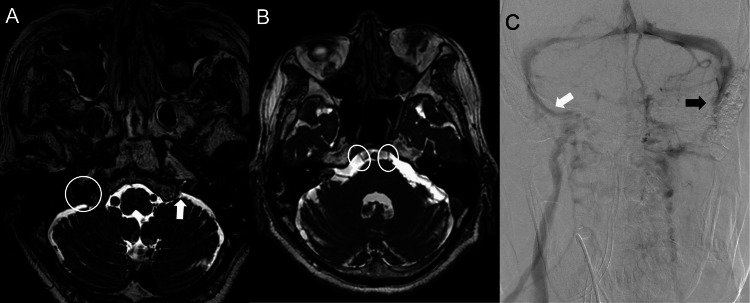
The heavily T2-weighted magnetic resonance imaging (A and B) and catheter-guided angiography (C) on hospital day two. A: White arrow indicates the left jugular foramen narrowed by the displaced fractured bone fragment, disrupting the lower cranial nerves running into the jugular foramen. White circle indicates the contralateral jugular foramen, which is slightly narrowed by the fractured bone fragment. B: White circles indicate the abducens nerves, which appear morphologically intact, and the structures around them are preserved. It implies that bilateral abducens nerve palsy was caused by stretching force at the time of injury. C: While the right internal jugular vein remains patent, the left sigmoid sinus (black arrow) is stagnant and the left jugular vein is almost unidentifiable. Blood flow in the sigmoid sinus drains from the emissary veins to the scalp veins.
